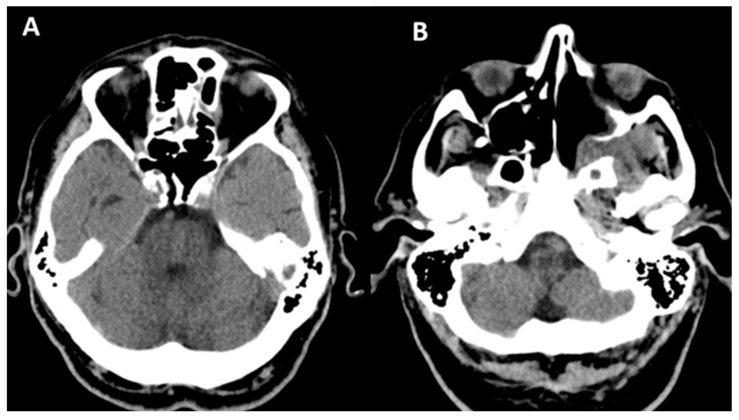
Cranial CT. (A,B) (scale bar equals 1.25 mm). Parafluid collection at the level of the left maxilla with osteolysis of the anterior, medial, and posterior walls; a similar aspect was observed at the level of the left sphenoidal sinus in its basal portion with osteolysis of the antero-inferior wall. Minimal thickening of the mucosa of the left frontal sinus and left ethmoidal cells, with the absence of the left nasal turbinate.
